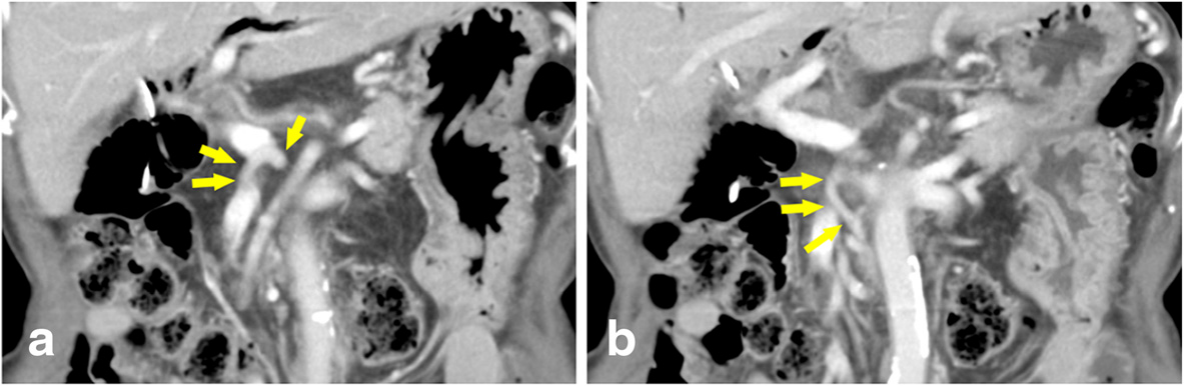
a, b Computed tomography image obtained 3 months postoperatively showing patency of the arc of Buhler
